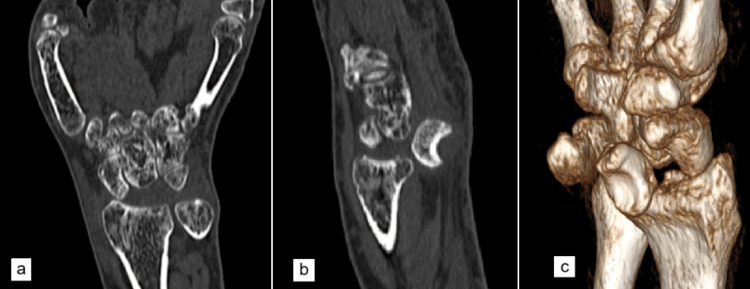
Representative CT images of the right wrist at the initial presentation showing (a) coronal section with absent lunate in the proximal carpal row, (b) sagittal section with the lunate dislocated into the carpal tunnel, and (c) three-dimensional reconstruction image of the wrist.
